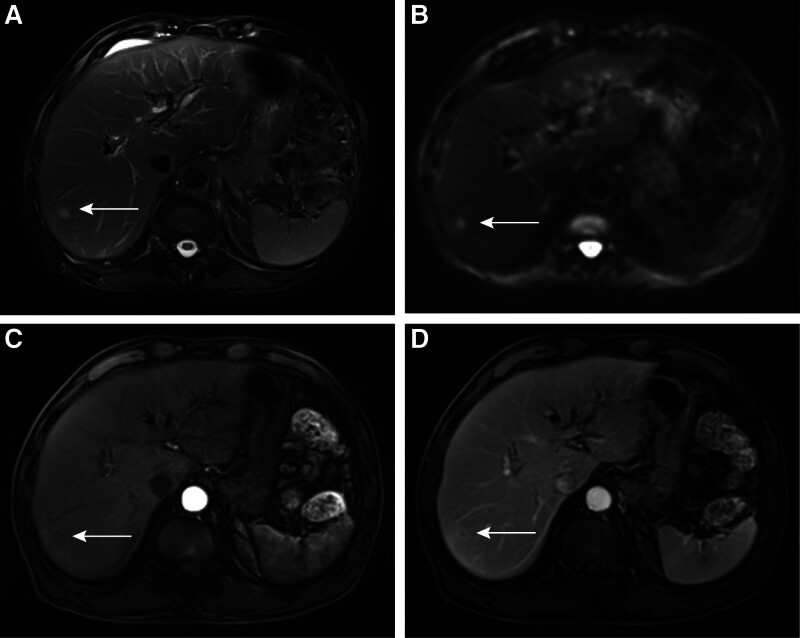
Abdominal enhanced MRI (6 mo postoperatively). T2-weighted imaging (A) and diffusion-weighted imaging (B) reveal a high-signal lesion approximately 4 × 5 mm in size in the right posterior liver lobe (indicated by the white arrow). (C) No significant enhancement is observed during the arterial phase of the enhanced scan (indicated by the white arrow). (D) During the portal venous phase of the enhanced scan, annular enhancement around the tumor is observed, consistent with a metastatic lesion (indicated by the white arrow). MRI = magnetic resonance imaging.
